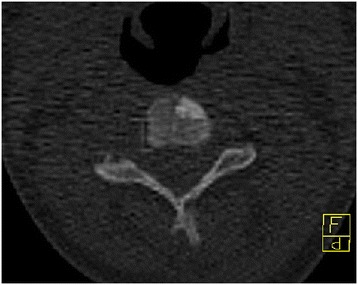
CT of cervical spine. Small sclerotic focus is located in the ventral part of C3 vertebral body - size 7 mm.
